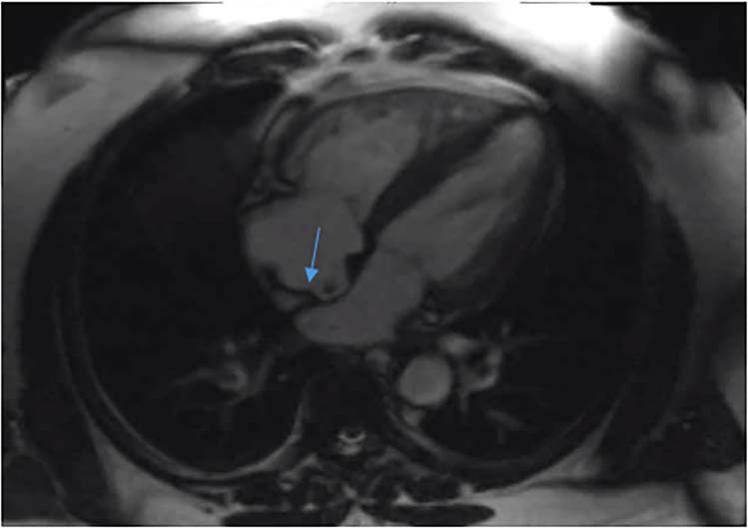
Cardiac magnetic resonance imaging showing a filling defect in the right atrium close to the interatrial septum.
